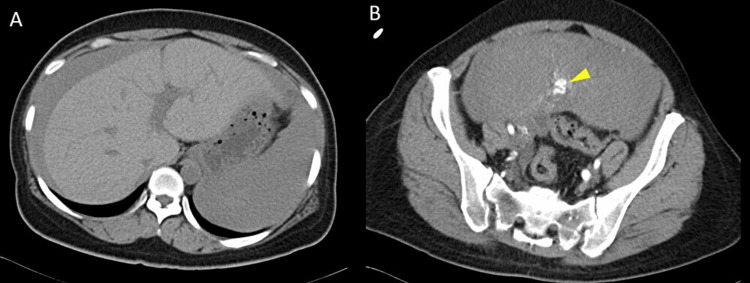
Contrast-enhanced computed tomography (CT). A: CT demonstrating a large volume of hemoperitoneum surrounding the liver; B: contrast-enhanced CT demonstrating a large uterine mass, presumed to be a leiomyoma, with prominent contrast-enhanced vessels visible along its surface, as indicated by the arrowhead.
